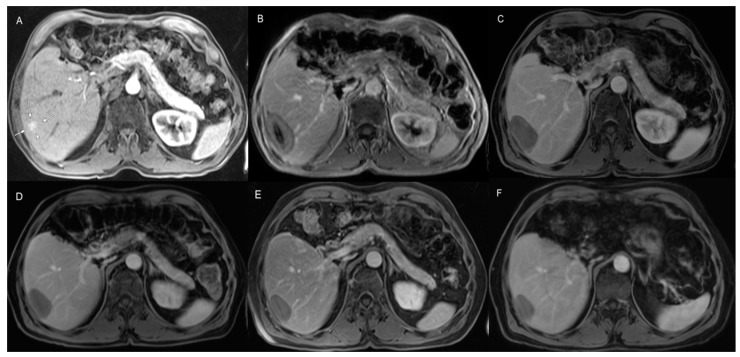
A 73-year-old male patient who developed intrahepatic recurrence 7 months after initially surgically resecting HCC. (A) pre-ablation contrast-enhanced MRI showed recurrent HCC lesion in liver segment 6 (white arrows). (B) A 24 h post-ablation MRI showed complete coverage of the recurrent tumor by the ablation area. (C) Three months post-ablation MRI. (D) Six months post-ablation MRI. (E) Twelve months post-ablation MRI. (F) Twenty-four months post-ablation MRI showed significant decrease in ablation area. This patient didn’t develop LTP or IDR during the follow-up period.
